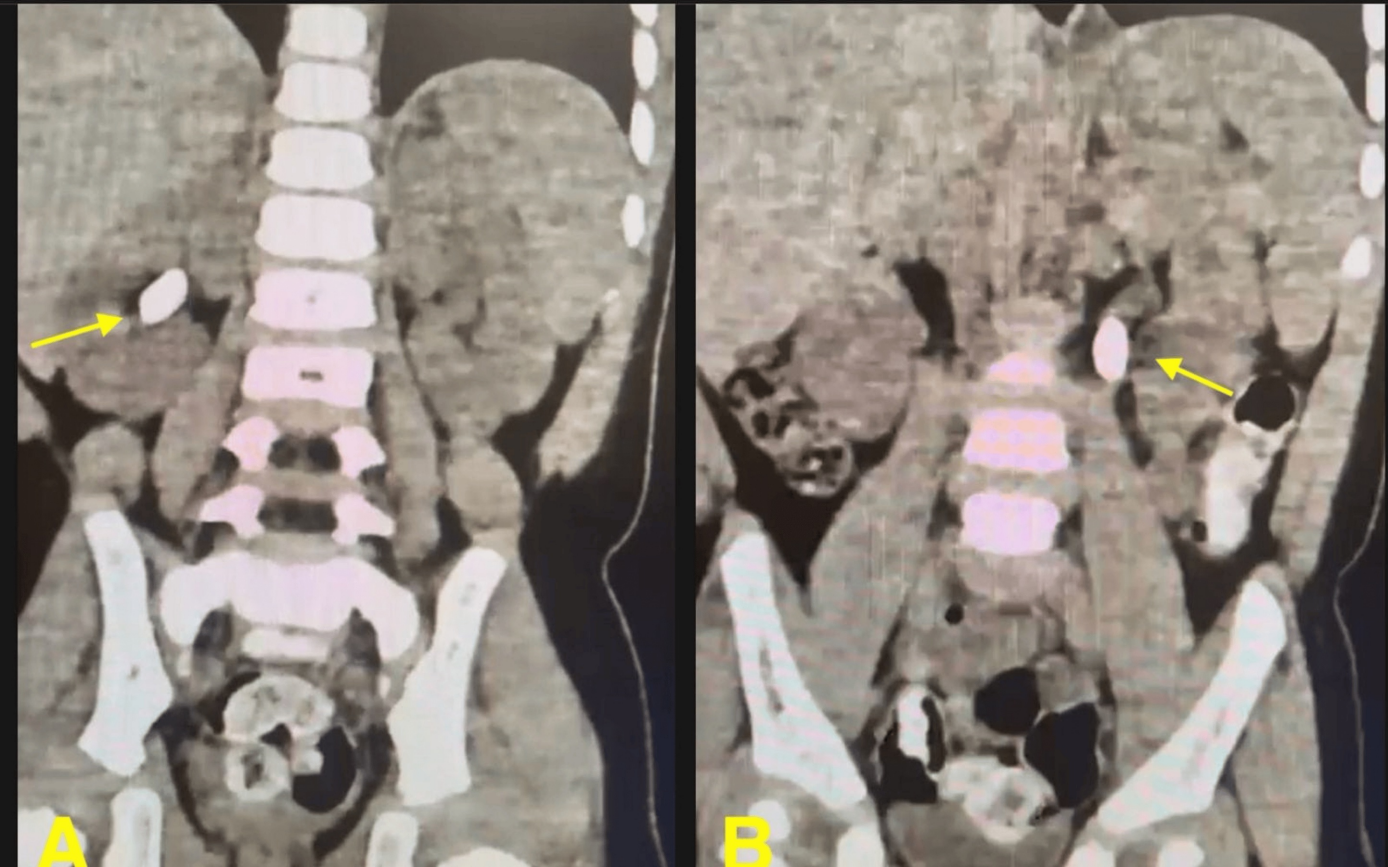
Coronal CT images demonstrating bilateral urolithiasis (A) Right renal stone (yellow arrow) located within the renal pelvis. (B) Left ureteral stone (yellow arrow) positioned within the ureter, causing partial obstruction CT: computed tomography
